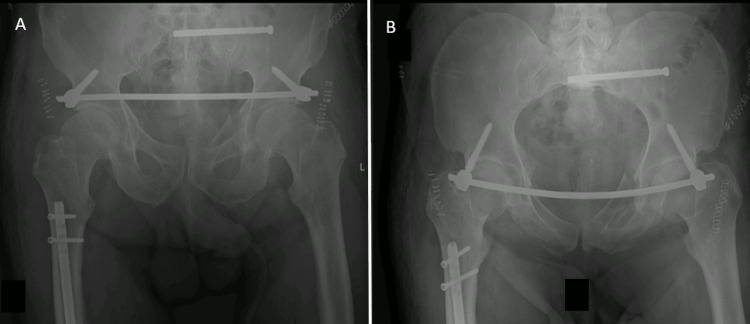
Anteroposterior (A) and inlet (B) radiographs of the pelvis showing the correct position of the INFIX and iliosacral screw INFIX - anterior pelvic internal fixator
